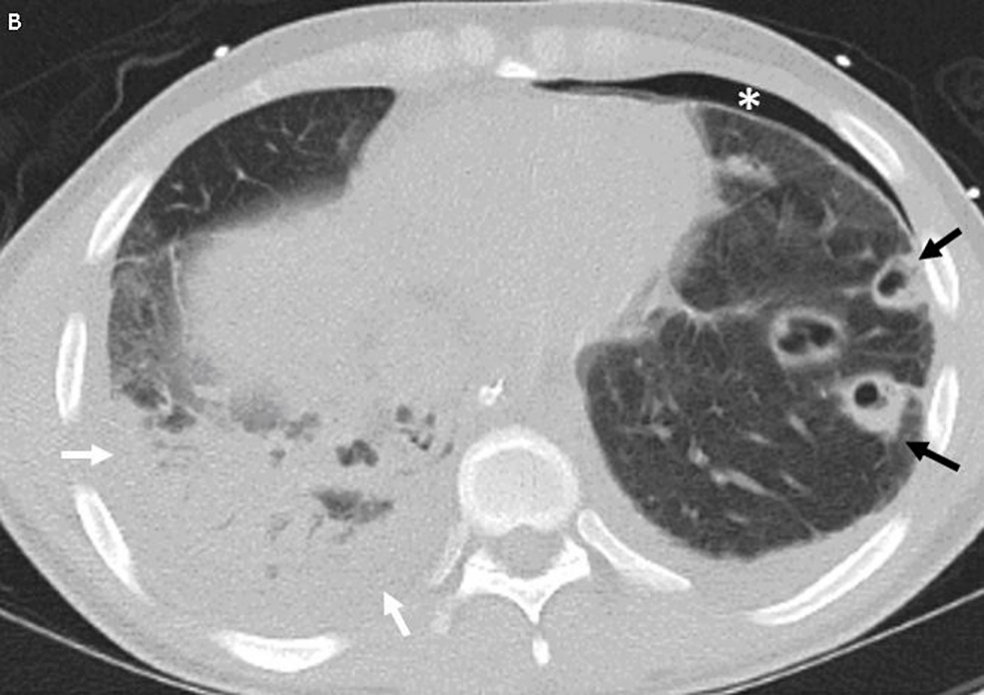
CT chest with IV contrast Select axial chest CT image of the same patient shows multiple cavitary septic embolic within the left lung (black arrows) along with a small pneumothorax (*) and small left pleural effusion. Airspace consolidation in the right lung (white arrows) corresponds to pneumonia with an adjacent small right pleural effusion CT: computed tomography; IV: intravenous
